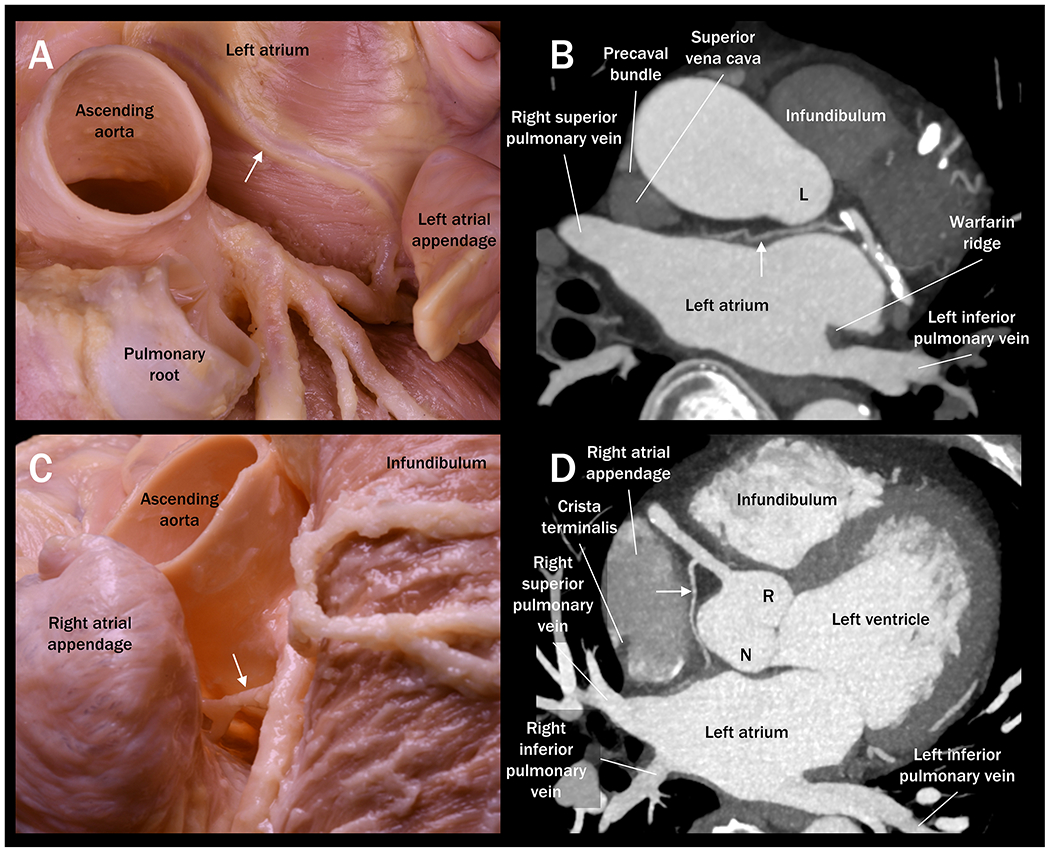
Anatomy of the left and right sinuatrial nodal arteries. (A) High-resolution dissection-enhanced photograph of the left sinuatrial nodal artery (white arrow), which originates from the left circumflex artery and runs along the anterior wall of the left atrium within the Bachmann’s bundle. (B) Multiplanar reconstruction image from the cardiac computed tomography shows the left sinuatrial nodal artery (white arrow) running similar course, suggesting a potential risk of injury of this artery during radiofrequency catheter ablation of the anterior wall of the left atrium. (C) High-resolution dissection-enhanced photograph shows the right sinuatrial nodal artery (white arrow) running between the right atrial appendage and the aortic root. Note that epicardial fat filling this region was thoroughly removed to expose this artery. (D) Multiplanar reconstruction image from the cardiac computed tomography shows the right sinuatrial nodal artery (white arrow) running similar course, suggesting a potential risk of injury of this artery during radiofrequency catheter ablation of the medial wall of the right atrial appendage. L: left coronary aortic sinus; N: non-coronary aortic sinus; R: right coronary aortic sinus.
